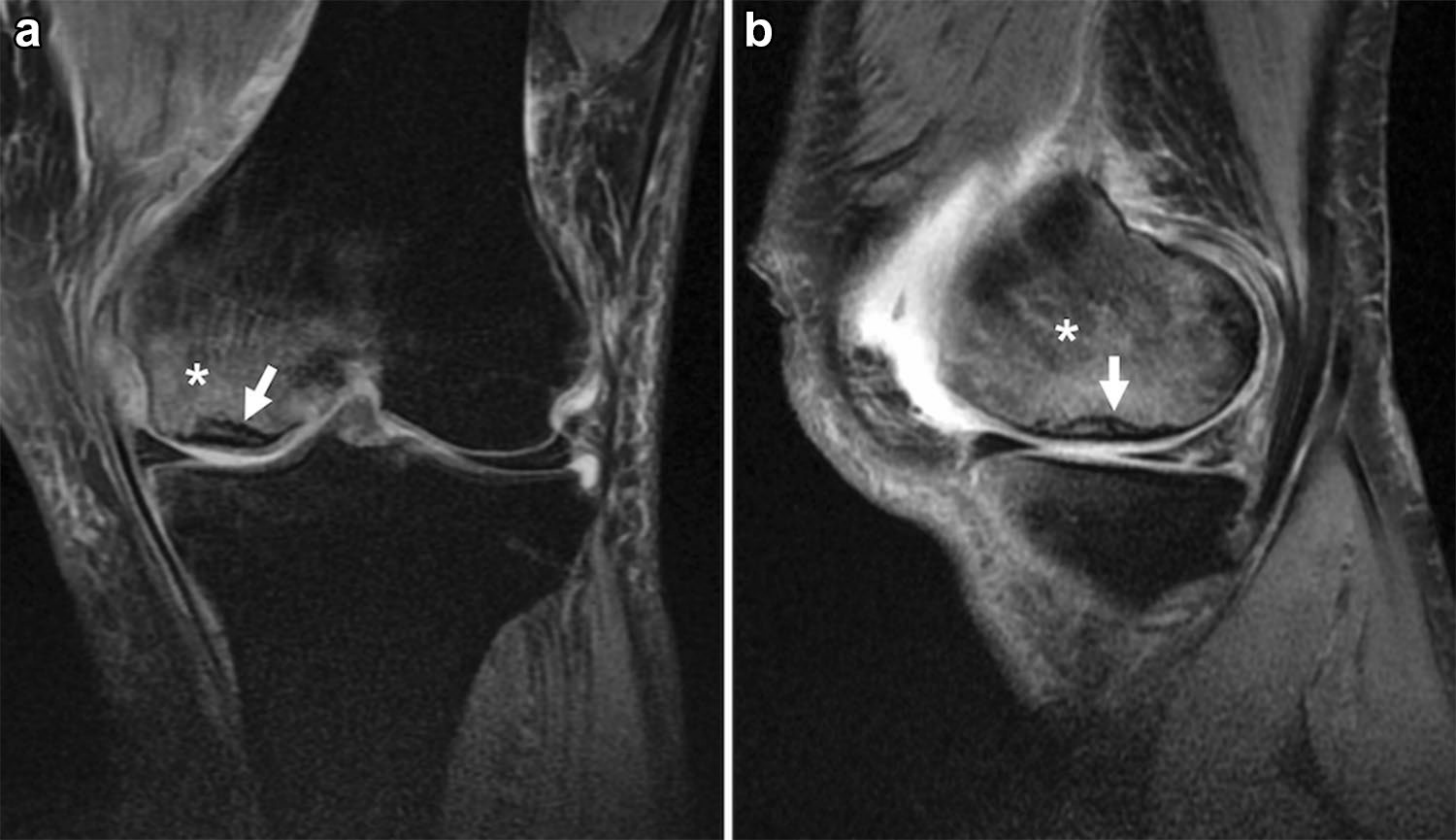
MRI of a 50-year-old man with a complaint of sudden left knee pain. a Coronal fat-suppressed proton density-weighted image (FS-PDWI), and b sagittal FS-PDWI show extensive bone marrow edema-like signal intensity over the medial femoral condyle (asterisk). A subchondral hypointense line is observed a few millimeters above the subchondral plate (arrow). Note that the line is almost parallel to the subchondral plate and open-ended in the medial part
